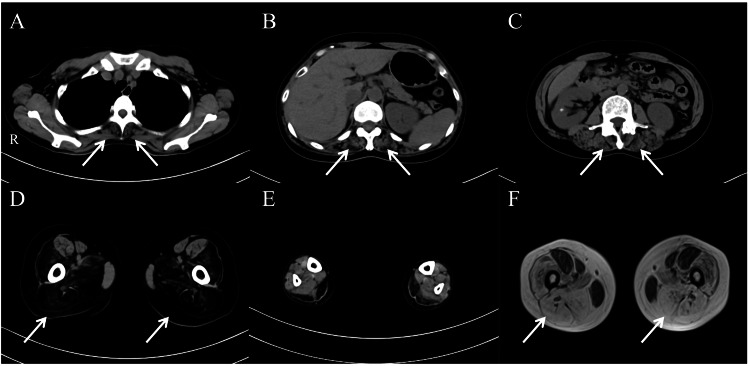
Muscle imaging findings in this case. (A-E) Muscle computed tomography findings. (F) Muscle magnetic resonance T2-weighted image at the thigh level (i.e., same level as in image D). The patient presents with atrophy of the paraspinal and dorsal thigh muscles and fat displacement of the thigh muscles, except for the rectus femoris, sartorius, and gracilis muscles.
